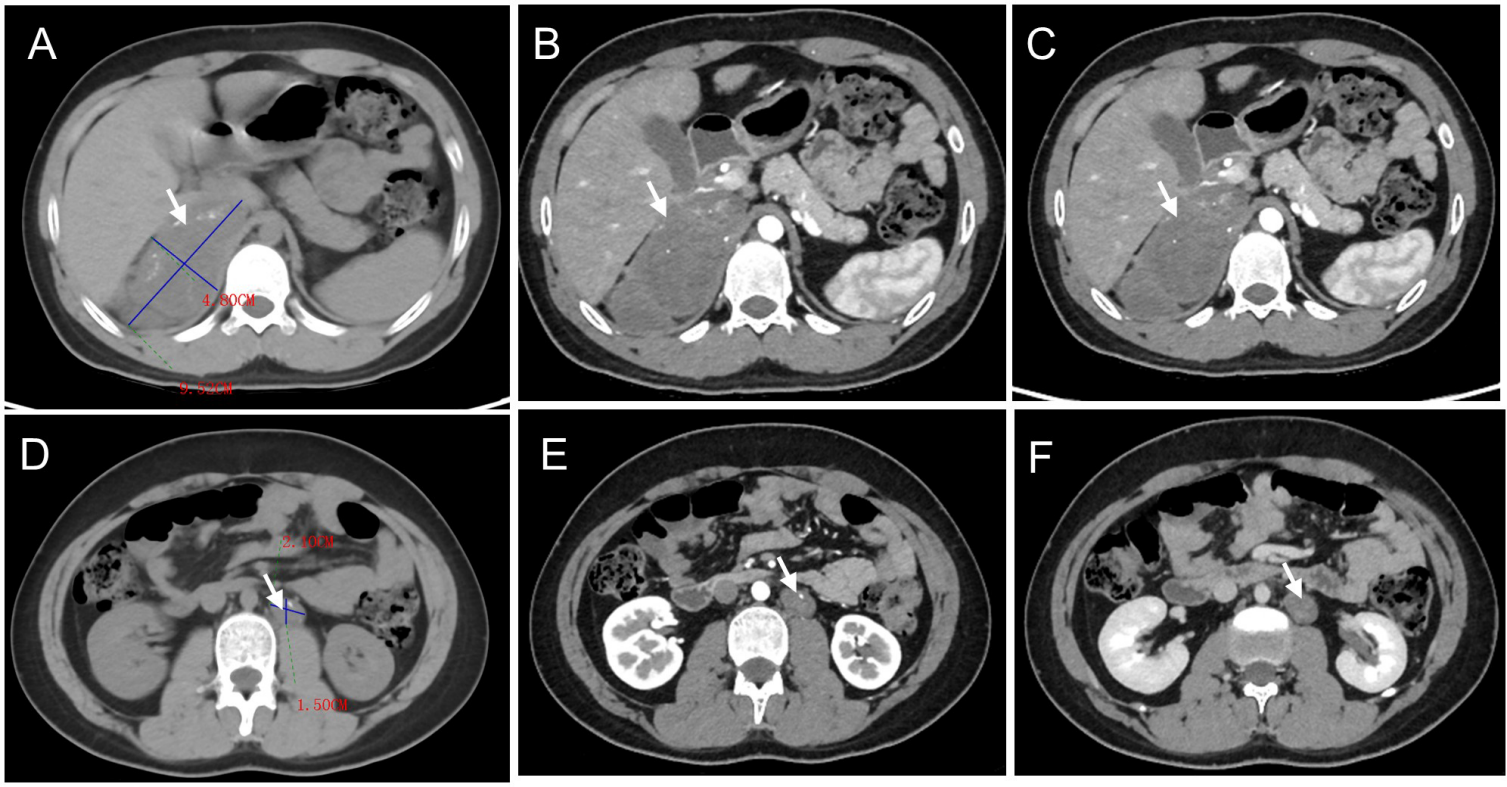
Abdominal CT findings of ganglioneuroblastoma in the same pediatric patient as depicted in Figure 1, involving the right (A-C) and left (D-F) retroperitoneal regions. On non-contrast CT scans ((A, D); lesions indicated by arrows), heterogeneous soft-tissue density masses were identified in the retroperitoneal space, with irregular calcifications observed in both cases. Contrast-enhanced CT scans demonstrated mild heterogeneous enhancement of the masses during the arterial phase ((B, E); arrows) and venous phase ((C, F); arrows).
